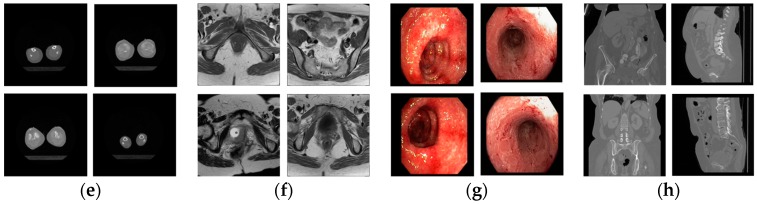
Selected example images for showing high inter-class similarity; (a,e) hip CT in Figure 5a-1 and knee CT in Figure 5a-2, respectively; (b,f) cervix MRI in Figure 5a-4 and bladder MRI in Figure 5a-9, respectively; (c,g) benign tumors in Figure 5d-7 and Rectum in Figure 5d-1, respectively; (d,h) urography CT in Figure 5b-2 and uterus CT in Figure 5b-5, respectively.
